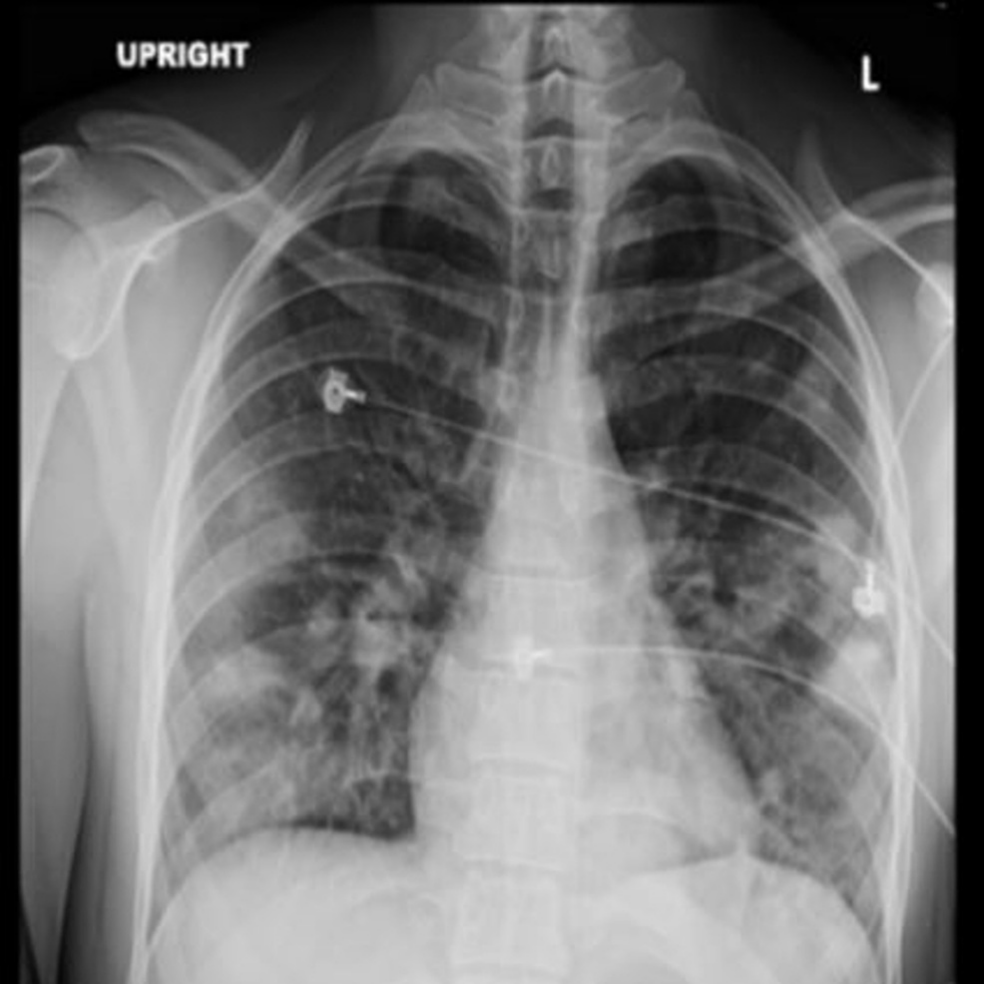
Chest X-ray Chest radiograph demonstrates multiple parenchymal opacities throughout both lungs in a peripheral distribution
